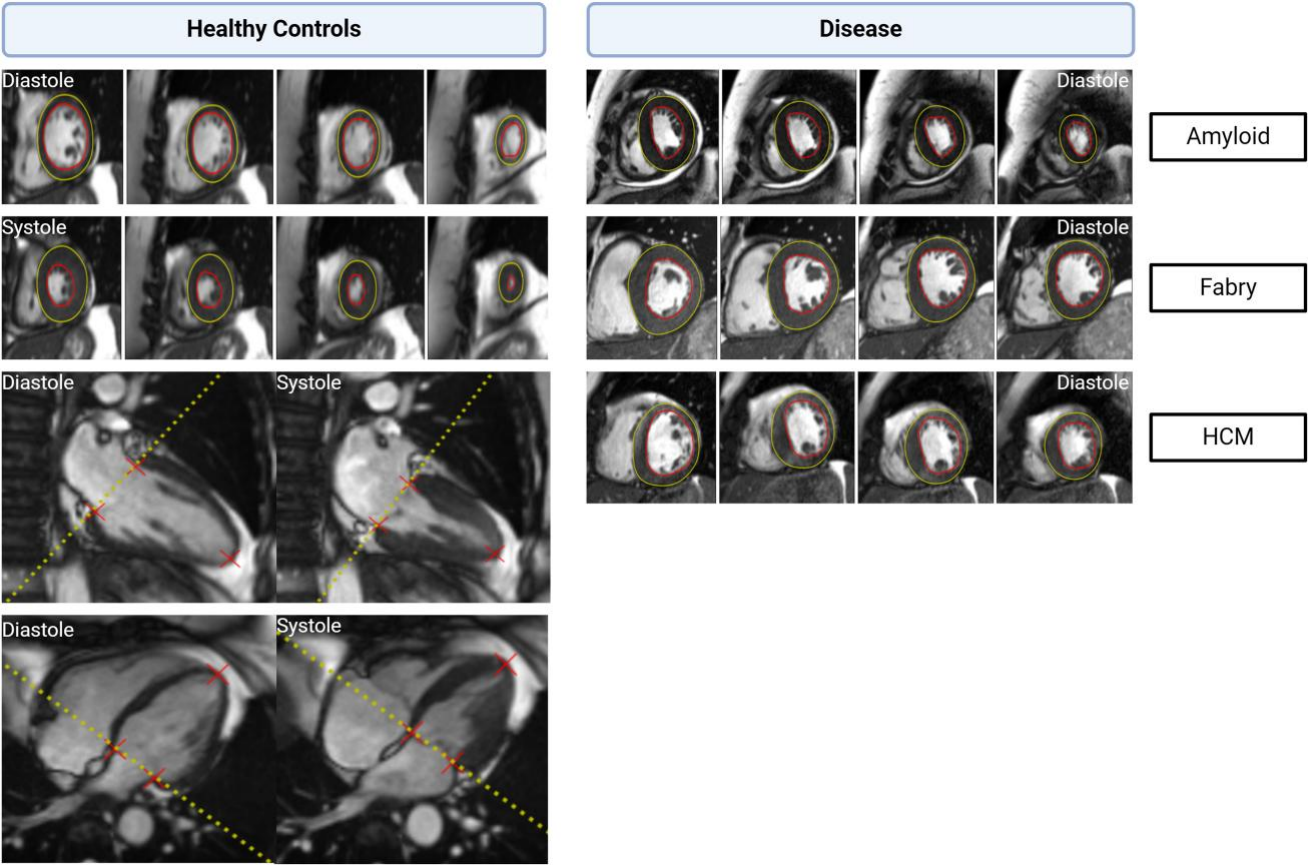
Automated AI-based myocardial segmentation in health and disease. Legend: Representative cardiac MRI images showing how the AI tool used in this study segmented the myocardium in healthy controls and example disease states. Left: Healthy controls in diastole and systole with normal myocardial contraction. Right: Pathological hypertrophy in TTR amyloidosis, Fabry disease, and hypertrophic cardiomyopathy (HCM), showing altered myocardial morphology and contraction patterns in diastole. Green and red contours indicate automated endocardial and epicardial segmentation, respectively. Red crosses mark key anatomical landmarks (mitral valve insertion points and apex) in long axis imaging used for alignment and consistency in myocardial segmentation across diastole and systole.
